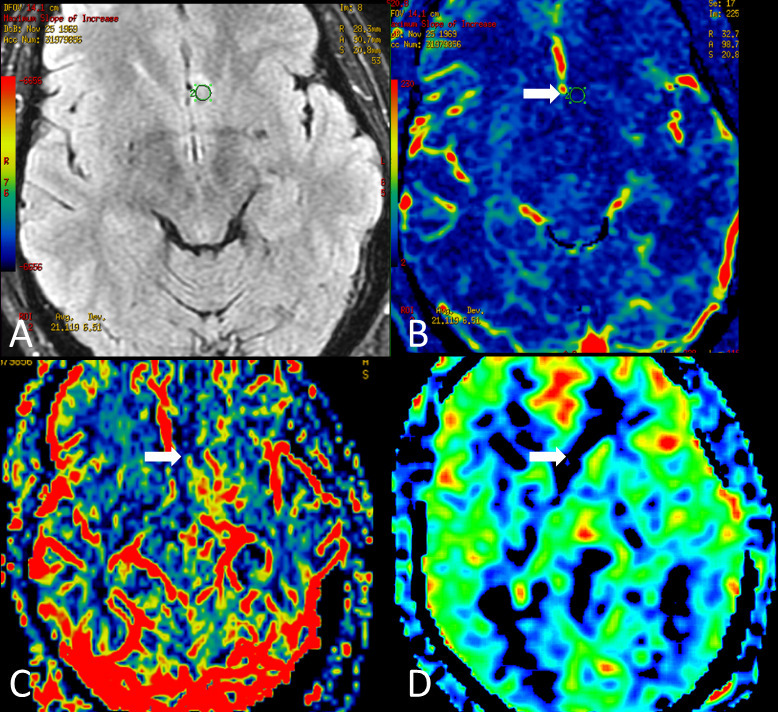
Perfusion MRI of the same patient. Axial FLAIR (A) localizes the tumour. Dynamic Contrast-Enhancement (DCE) permeability map (B) shows a standard map of Maximum Slope of Increase. Dynamic susceptibility contrast (DSC) rCBV and Arterial Spin Labeling CBF maps (C, and D, respectively) demonstrate normal capillary density and cerebral blood flow, compatible with a hypoperfused lesion.
